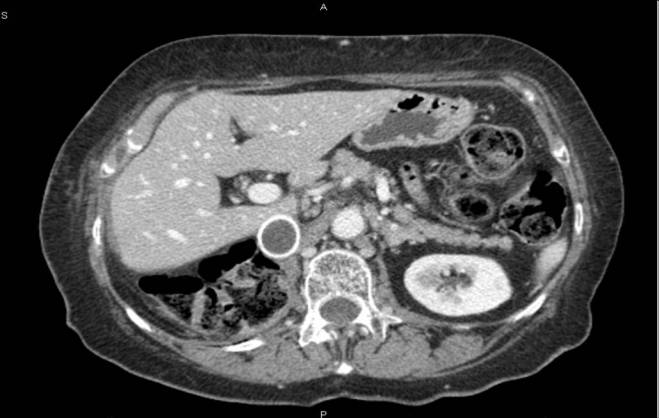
Post-operative CT scan—patient 8
